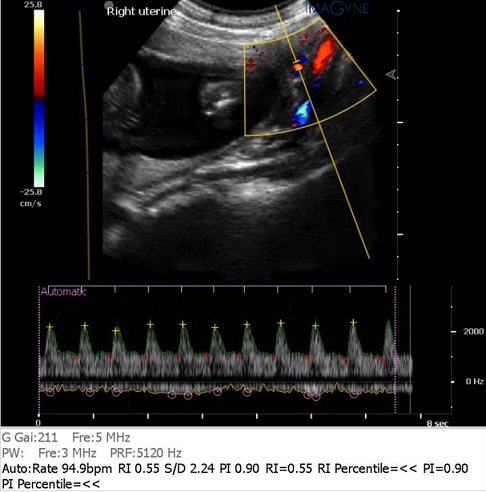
Doppler waveform/pattern
